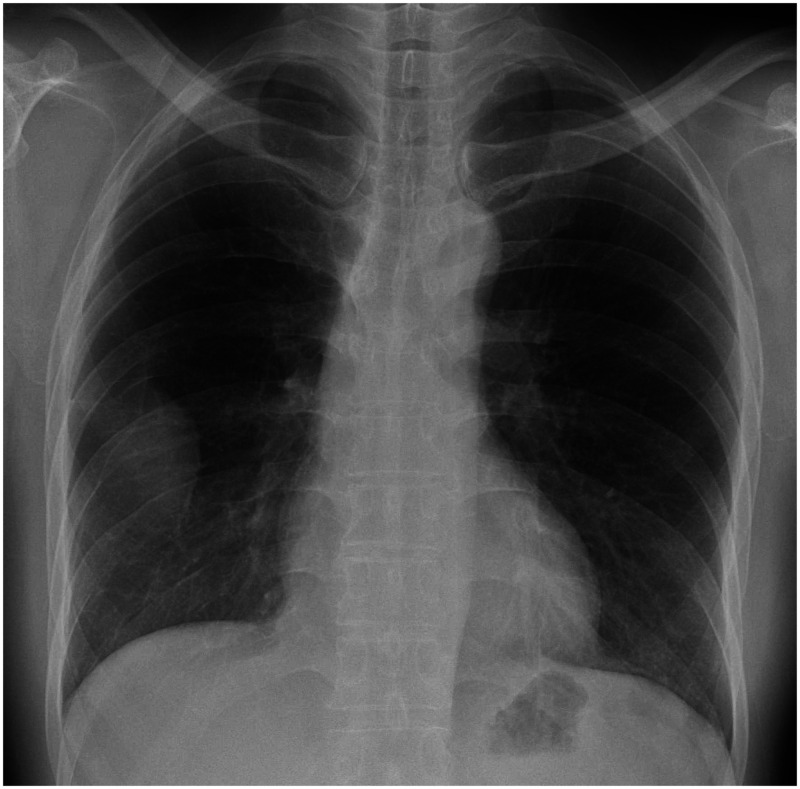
Incomplete border sign on chest X-ray. A mass was observed in the right lower lung field. Note that the outline of the mass disappeared on the right side (incomplete border sign), suggesting an extra-pulmonary lesion.
